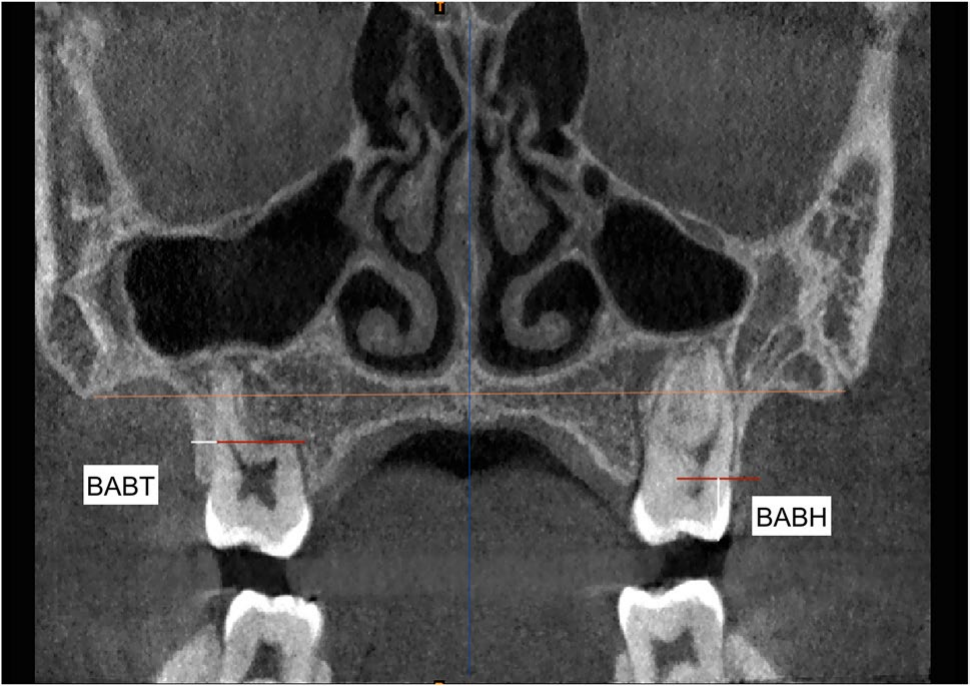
Buccal alveolar bone thickness (BABT) and buccal alveolar bone height (BABH) variables representing the characteristics of the buccal alveolar bone plate assessed on the coronal view of the CBCT scans
